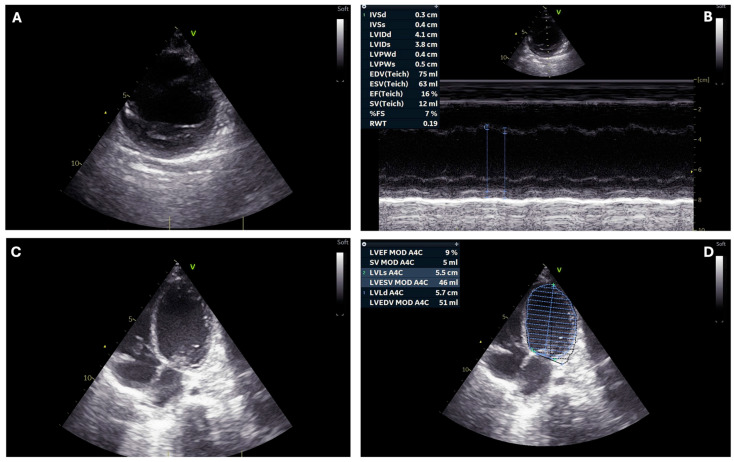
Echocardiography from patient 4. Parasternal short axis (panel A) and four-chamber (panel C) views show severe dilatation of the left ventricle. Ventricular hypokinesia was assessed by means of M-Mode (panel B) and Biplane Simpson Method (panel D).
